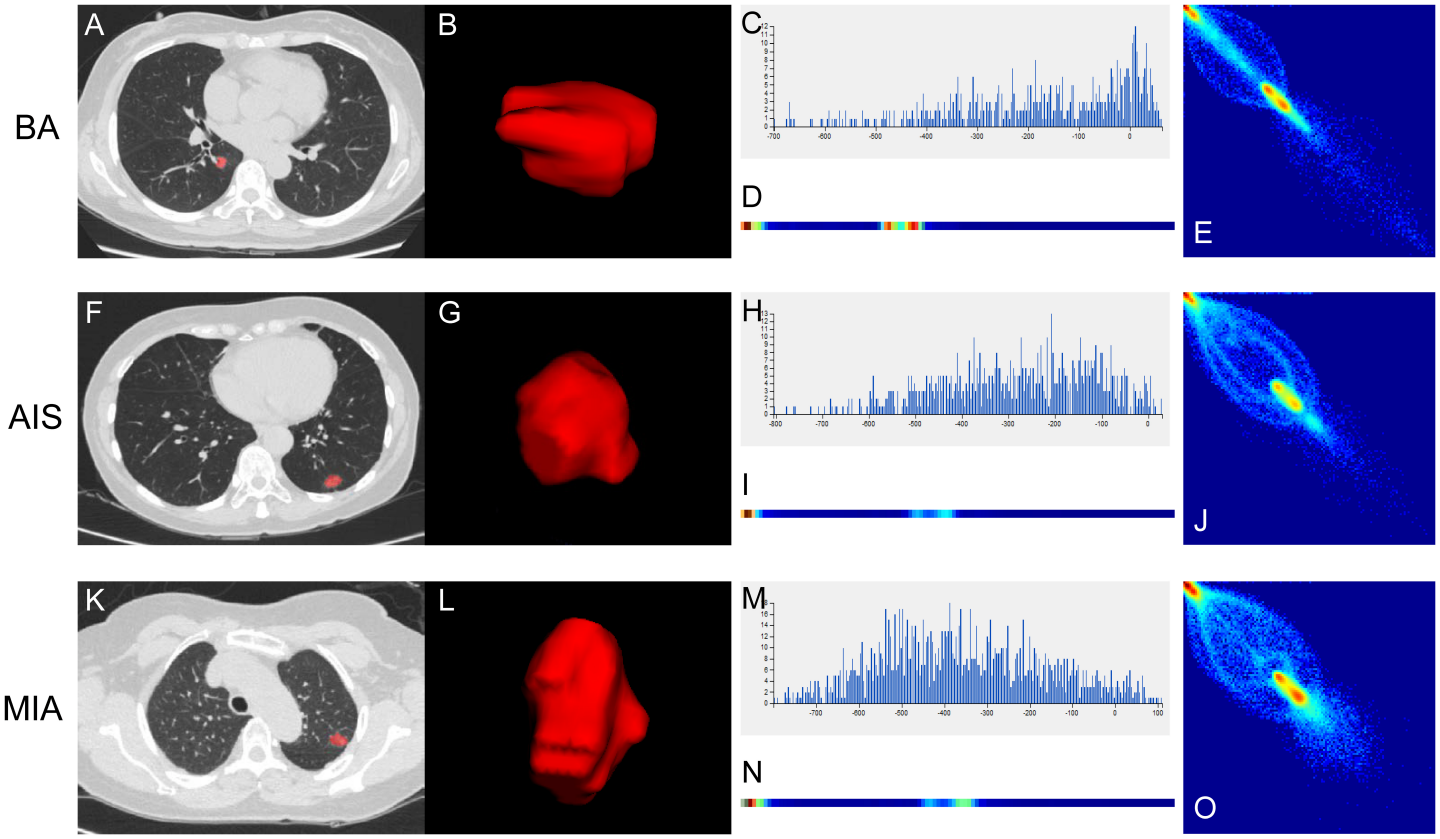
Three representative sets of CT texture features of patients with BA (A–E), AIS (F–J), and MIA (K–O). (A,F,K) Thin-section unenhanced CT images. (B,G,L) VOIs delineated by the ITK-SNAP software. (C,H,M) Histograms of texture parameters of the three lesions showed a marked difference. The distribution of (H) and (M) were more dispersed than the distribution of (C). (D,I,N) GLRLM features of the three lesions. The frequency of grayscale changes of (D) was more stable than that of (I,N). (E,J,O) GLCM features of the three lesions. The distribution of (E) was more concentrated than the distribution of (J,O).
